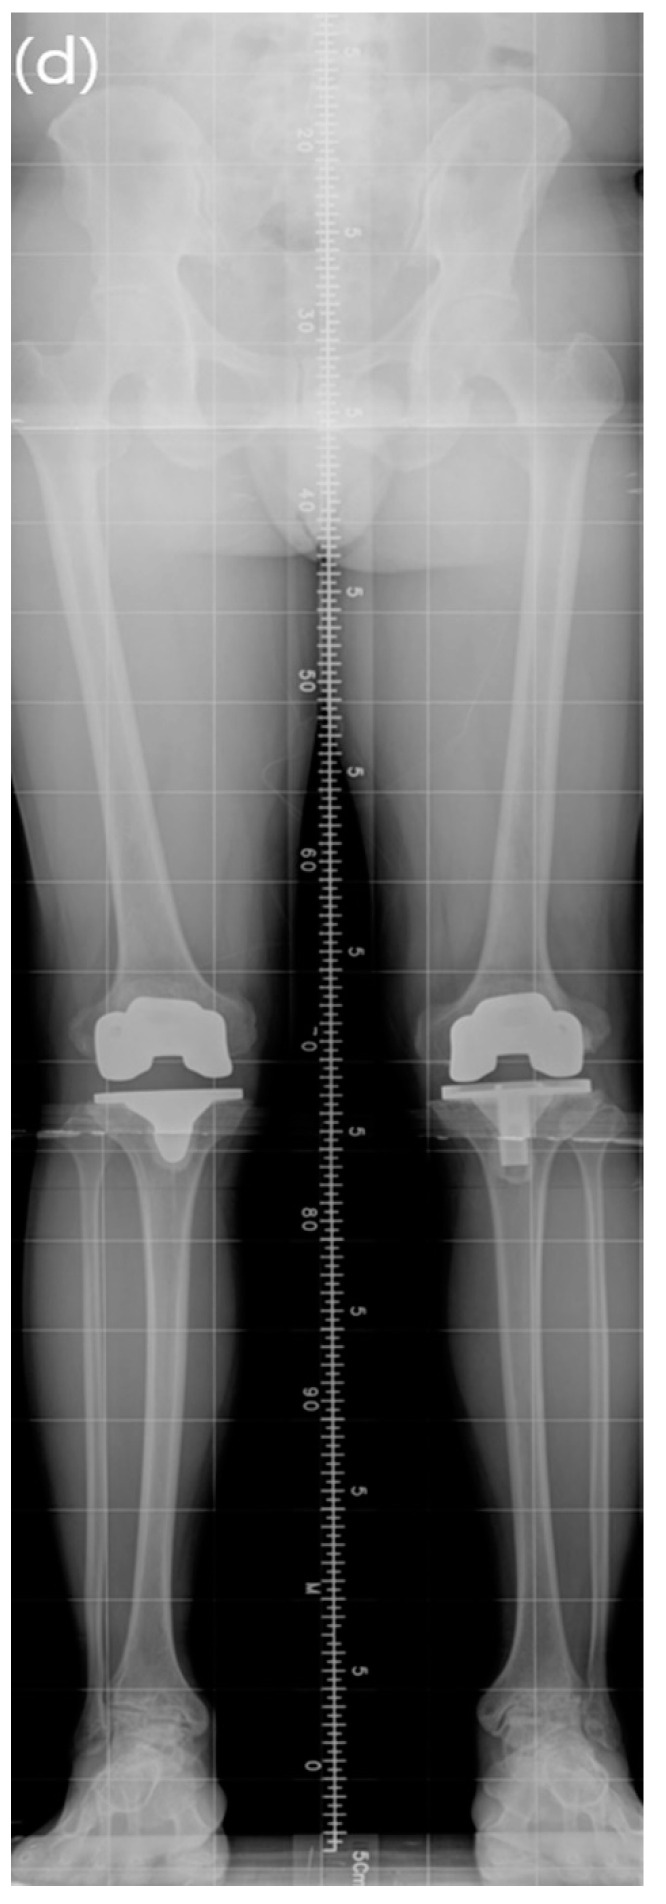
A 36-year-old hemophiliac male with severe valgus deformity. Flexion contractures of both knees were 10° on the right and 15° on the left, respectively. The range of motion (ROM) in both knees was limited to 120°. (a) Preoperative standing anteroposterior and lateral radiographs. (b) A preoperative lower-extremity orthogram showed 15° valgus of the right lower limb and 14° valgus of the left lower limb. (c) A postoperative radiograph taken at 11 years after surgery showed well-positioned components. (d) A postoperative lower-extremity orthogram taken at 11 years after surgery revealed neutral alignment in both knees. The ROM of both knees was improved to 140° without flexion contracture.
